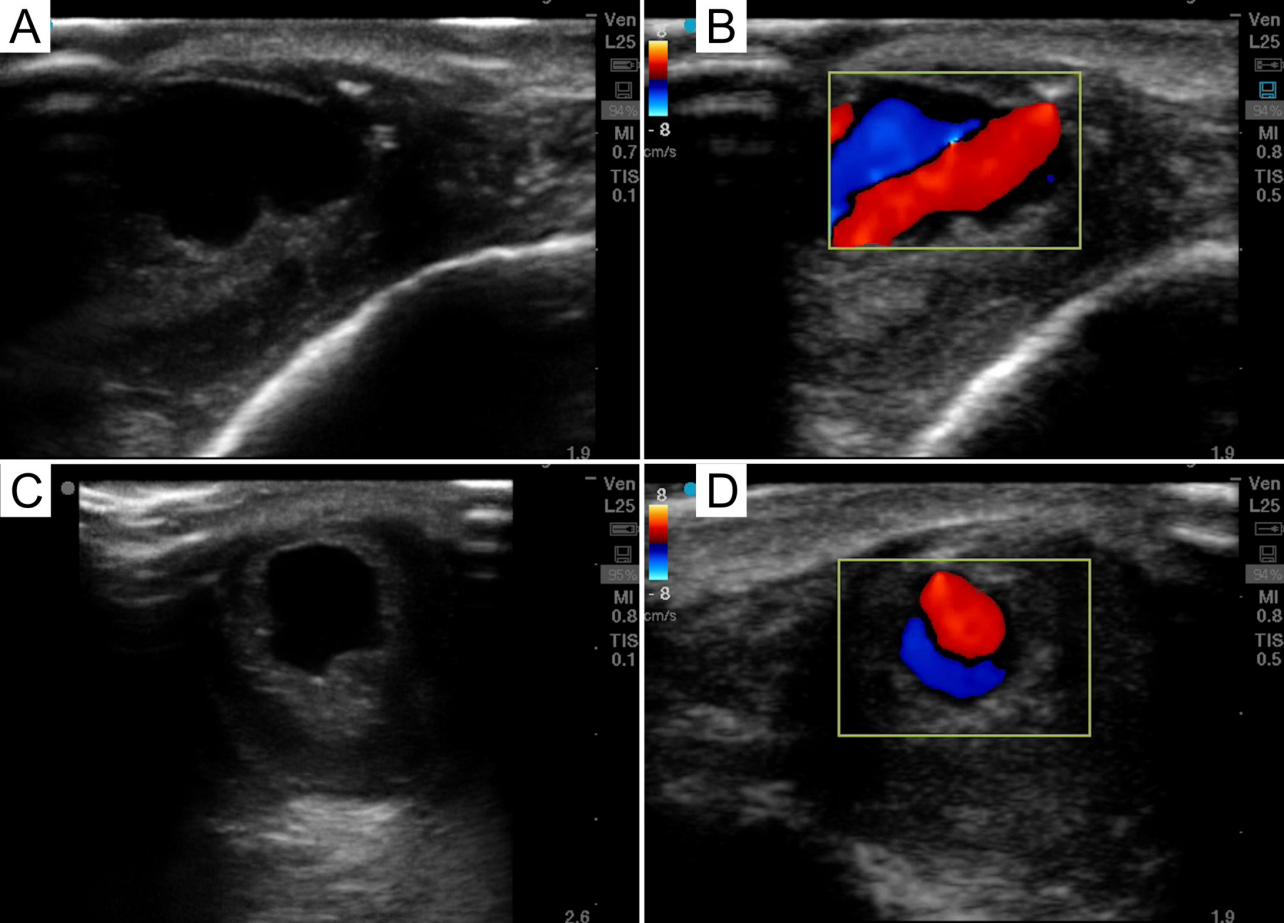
A, B are long axis POCUS images of the right forehead soft tissue mass (B with color Doppler). C, D are short axis POCUS images (D with color Doppler). B and D demonstrate the“Ying-Yang” or “Pepsi” sign, which is due to bidirectional or turbulent blood flow.
